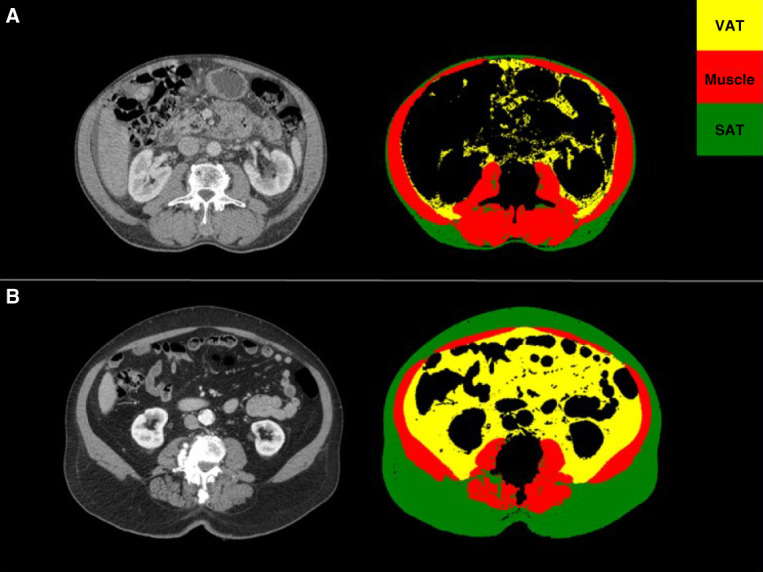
Measured and eGFR in two patients with body composition parameters assessed by CT scan. Skeletal muscle area is shown in red, VAT in yellow, and SAT in green. (A) A 68-year-old man with a normal BMI (23.8 kg/m^2^) and normal SMI (64.5 cm^2^/m^2^). Serum creatinine was 1.1 mg/dl; serum Cys C was 1.2 mg/L. His mGFR was 67 ml/min per 1.73 m^2^; eGFRcr was 73 ml/min per 1.73 m^2^; eGFRcys was 59 ml/min per 1.73 m^2^; eGFRcr-cys was 68 ml/min per 1.73 m^2^. (B) A 77-year-old man with a high BMI (39.9 kg/m^2^) and normal SMI (66.7 cm^2^/m^2^). Serum creatinine was 1.3 mg/dl; serum Cys C was 1.4 mg/L. His mGFR was 61 ml/min per 1.73 m^2^; eGFRcr was 57 ml/min per 1.73 m^2^; eGFRcys was 46 ml/min per 1.73 m^2^; eGFRcr-cys was 53 ml/min per 1.73 m^2^. GFR was estimated using the 2021 CKD-EPI equations. Sarcopenia is defined by a SMI <55 cm^2^/m^2^ for men. BMI, body mass index; CT, computed tomography; eGFRcys, eGFR based on the serum level of cystatin C; SAT, subcutaneous adiposity tissue; SMI, skeletal muscle index; VAT, visceral adiposity tissue.
